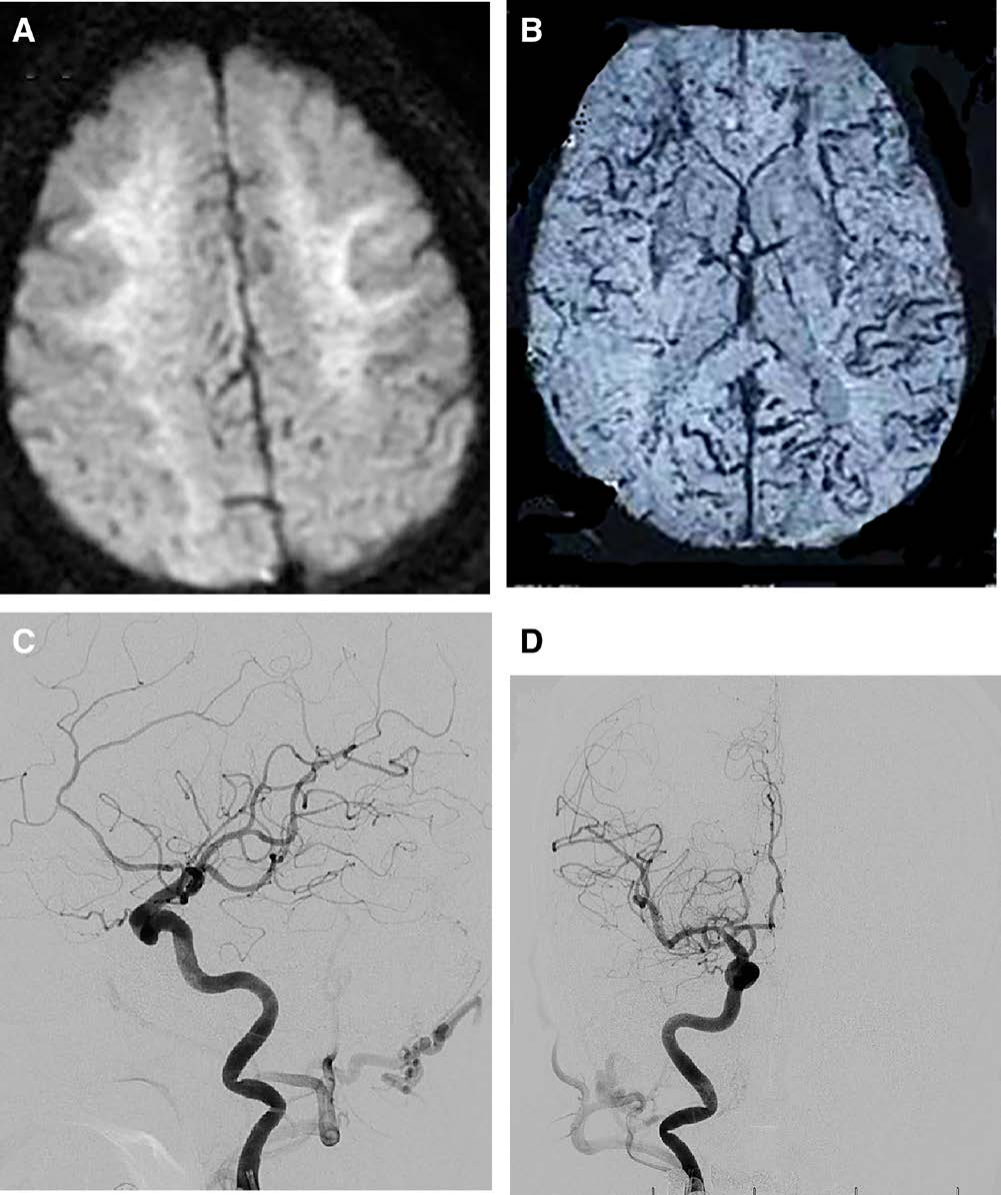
(A) Brain magnetic resonance imaging (MRI) indicated extensive white matter lesions in both cerebral hemispheres. (B) Craniocerebral susceptibility weighted imaging (SWI) indicated alterations in venous dilation. (C) Digital subtraction angiography (DSA) indicated that the upper and lower sagittal sinuses and bilateral transverse sinuses were affected. (D) DSA indicated that the upper and lower sagittal sinuses and bilateral transverse sinuses were affected.
